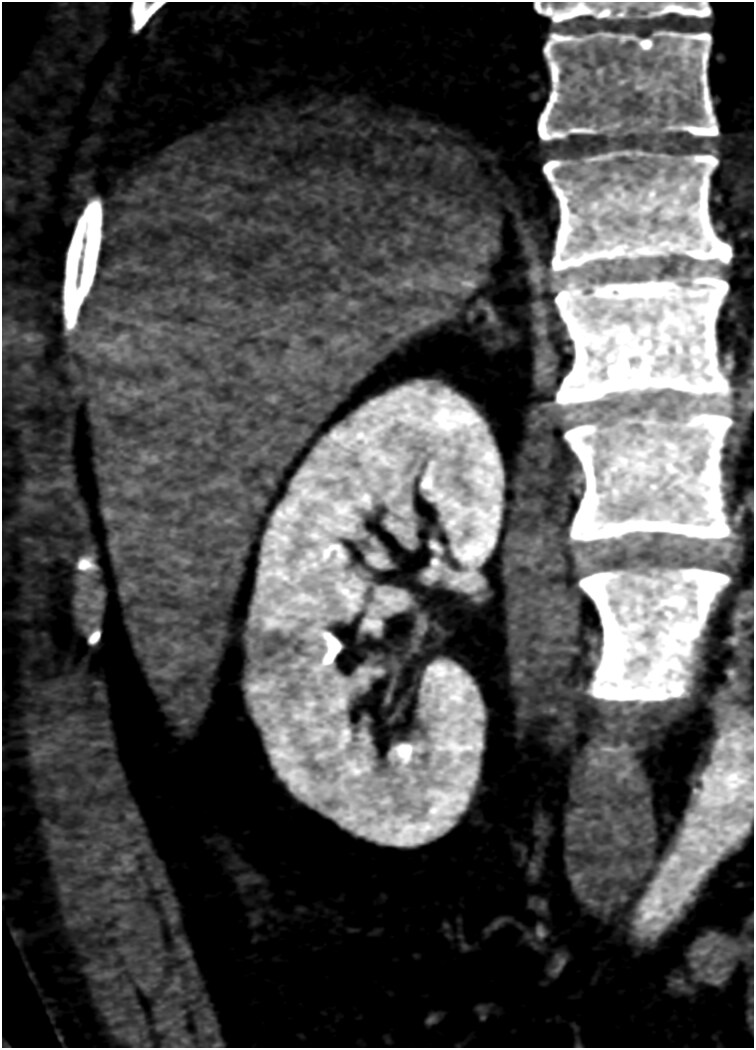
CT Abdo Pelvis demonstrating a right renal infarct.
